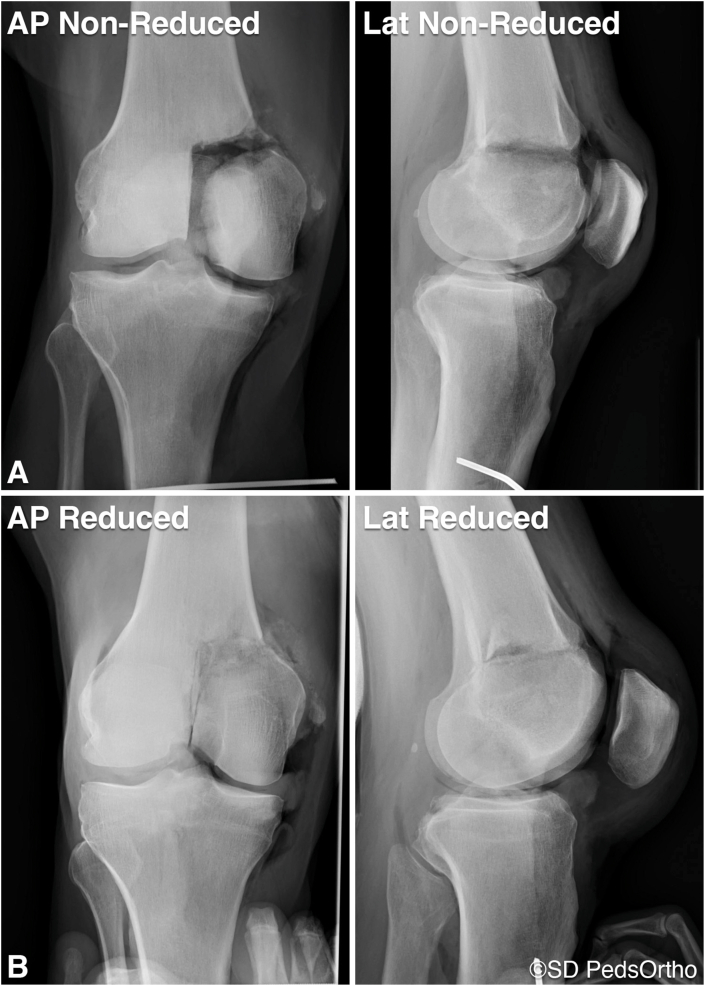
Radiographic images of (A) SH-III fracture and (B) post-reduction images. *SH-III, Salter-Harris type III.*
